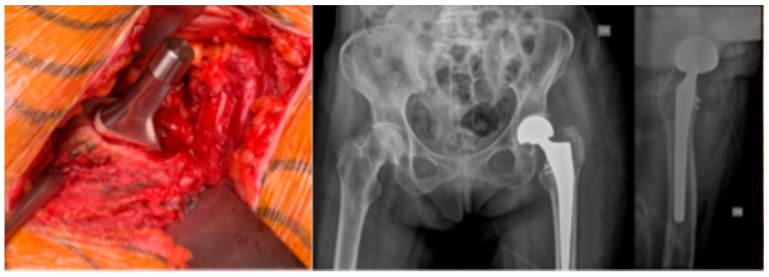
Intraoperative image showing an intraoperative periprosthetic proximal femoral fracture at the calcar level, fixed with two 2.7 mm screws, along with its postoperative X-rays at 6 months.
